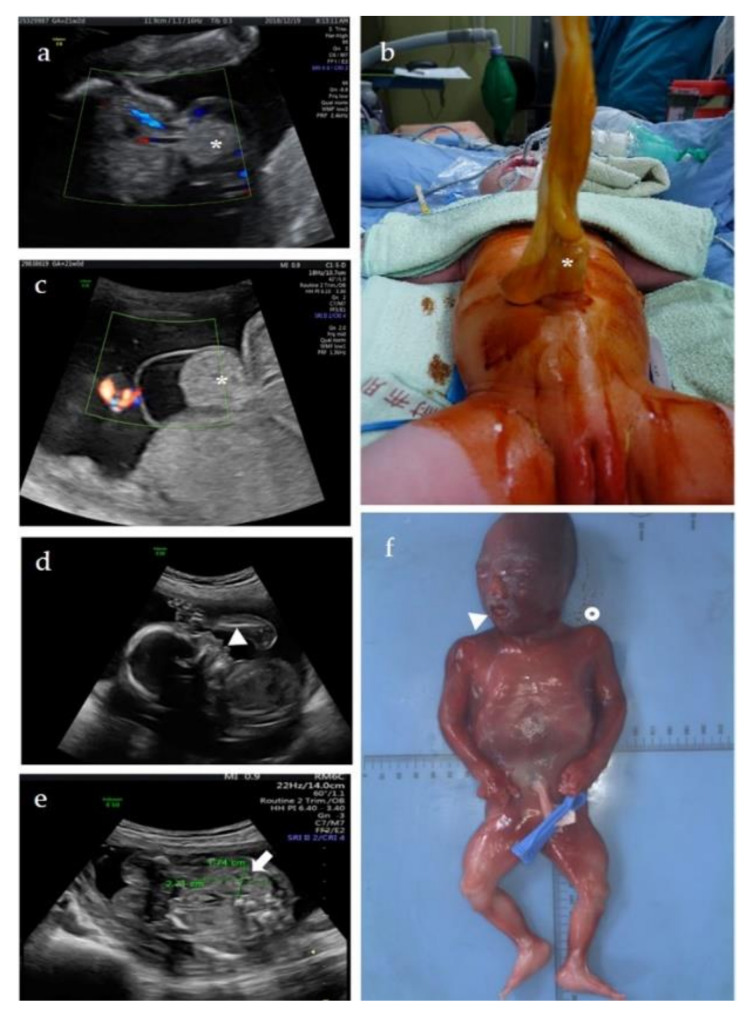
Clinical features of three patients with BWS. Patient 1 (a,b): Prenatal ultrasonography identified (a) isolated umbilical hernia (star) at GA = 21 weeks and two days. The position of cord insertion was normal and protruding small intestine was noted. (b) The umbilical hernia with 3 cm abdominal wall defect and small bowel protruding (star) was identified after birth (GA = 38 weeks). Macrosomia with body weight of 3850 g (96th percentile) was also noted. Patient 2 (c): Prenatal ultrasonography identified (c) isolated omphalocele (star) at GA = 21 weeks. The cord inserted on the apex of herniated sac. Patient 3 (d–f): Prenatal ultrasonography identified (d) protruding tongue (triangle) and (e) unilateral nephromegaly (fetal kidney length: 2.71 cm and transverse diameter: 1.74 cm; both values > 95th percentile) (arrow) at GA = 20 weeks and two days. (f) Macroglossia (triangle), board nose, and hemihyperplasia (circle) were further identified after TOP at GA = 21 weeks and 3 days.
